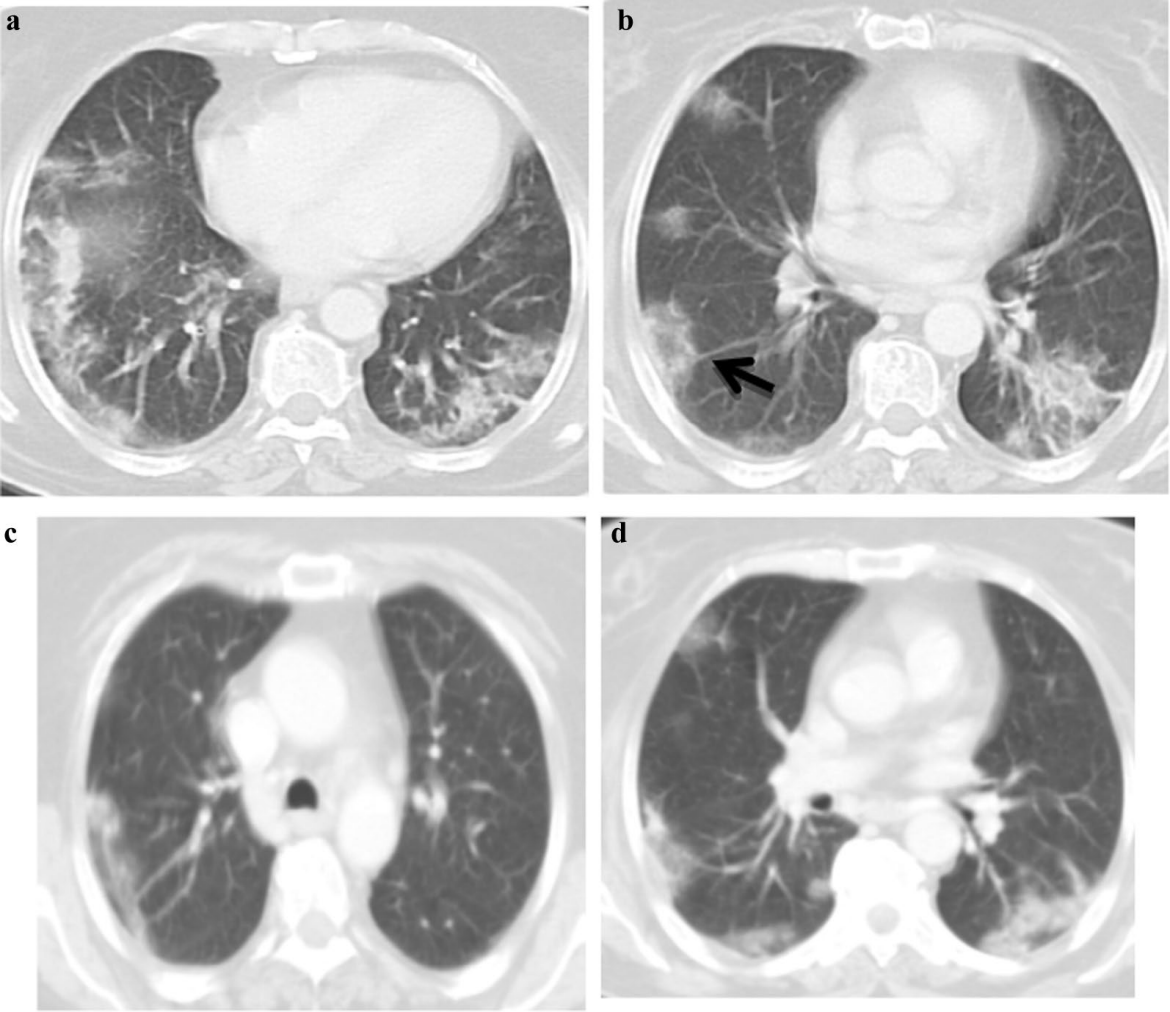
A 52-year-old female known history of cancer colon presented with anosmia, cough, fever, diarrhea and myalgia at September 2020 and positive PCR for COVID 19 infection, a and b post-contrast CT axial cuts shows bilateral patchy subpleural ground glass opacities with right lower lobe Atoll sign (arrowed). The patient diagnosed as COVID 19 infection with CT score severity: 10. Second infection dated March 2021 the patient presented with fever, cough, respiratory distress and myalgia with positive PCR test for COVID 19 infection. c and d postcontrast CT chest images show bilateral patchy subpleural ground glass opacities. The patient diagnosed as COVID 19 re-infection with CT severity score: 4
